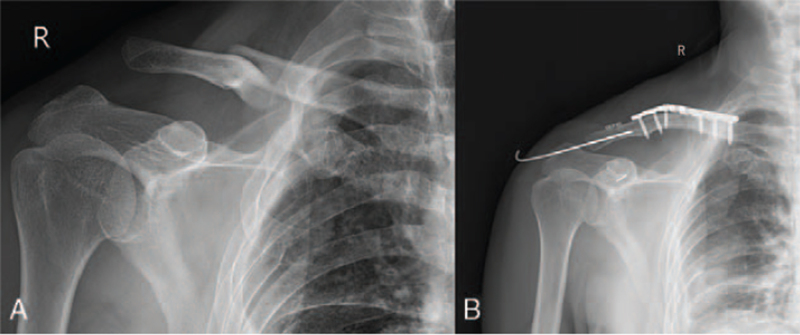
A. Anteroposterior digital radiography (DR) of the right shoulder shows mid-third clavicle fracture with ipsilateral type V ACJ dislocation (according to Rockwood classification). B. The postoperative radiograph showing anatomical reduction in the ACJ. ACJ = acromioclavicular joint.
